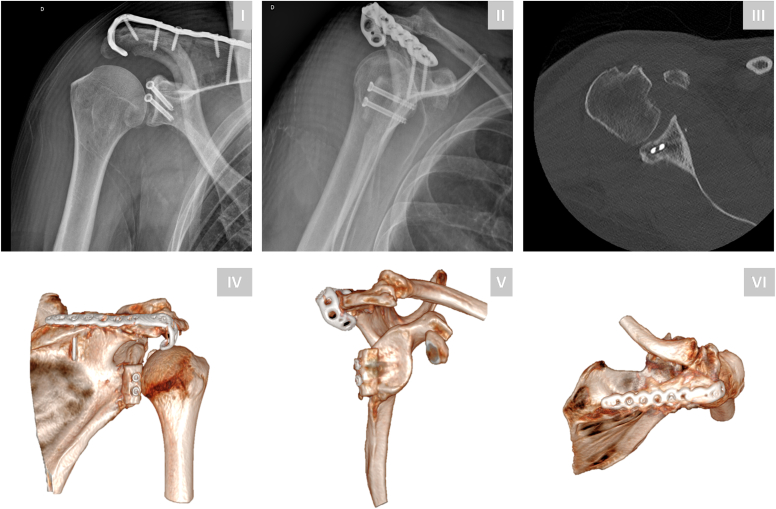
Postoperative imaging following open reduction, posterior glenoid bone block placement, and plate fixation of the acromion fracture. Standard anteroposterior and lateral radiographs (I-II) demonstrate appropriate reduction and implant placement. Axial CT imaging (III) and 3D reconstructions (IV-VI) confirm reduction and centering of the humeral head. *CT*, computed tomography; *3D*, three-dimensional.
